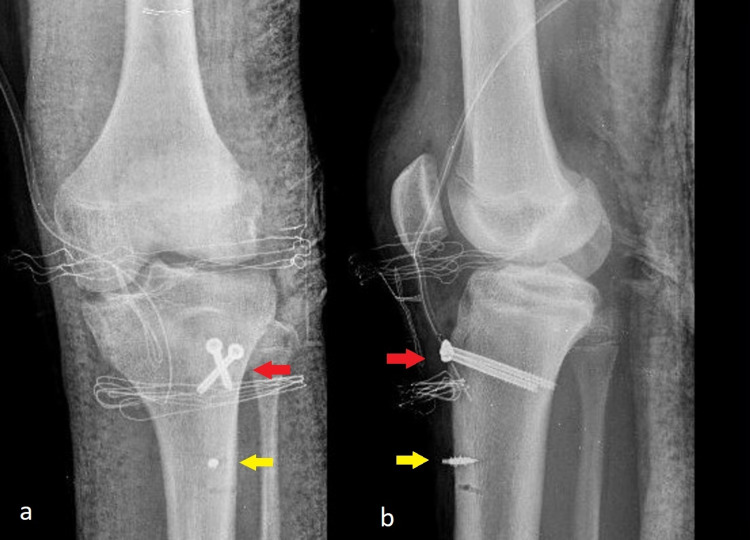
Immediate postoperative AP (a) and lateral (b) radiographs The two cannulated screws (red arrows) and the 5 mm anchor below the tibial tubercle (yellow arrows) are noted. AP - anteroposterior
